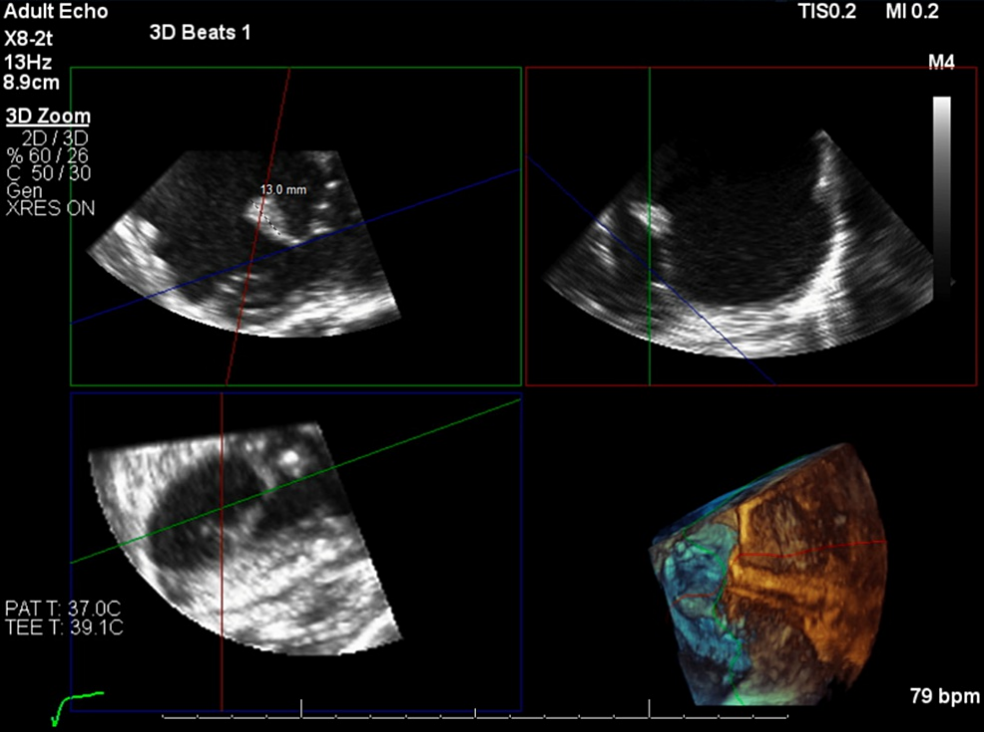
TEE showing a flail, anterior tricuspid valve leaflet with attached septic vegetation (13.0 mm measured structure). TEE, transesophageal echocardiogram
